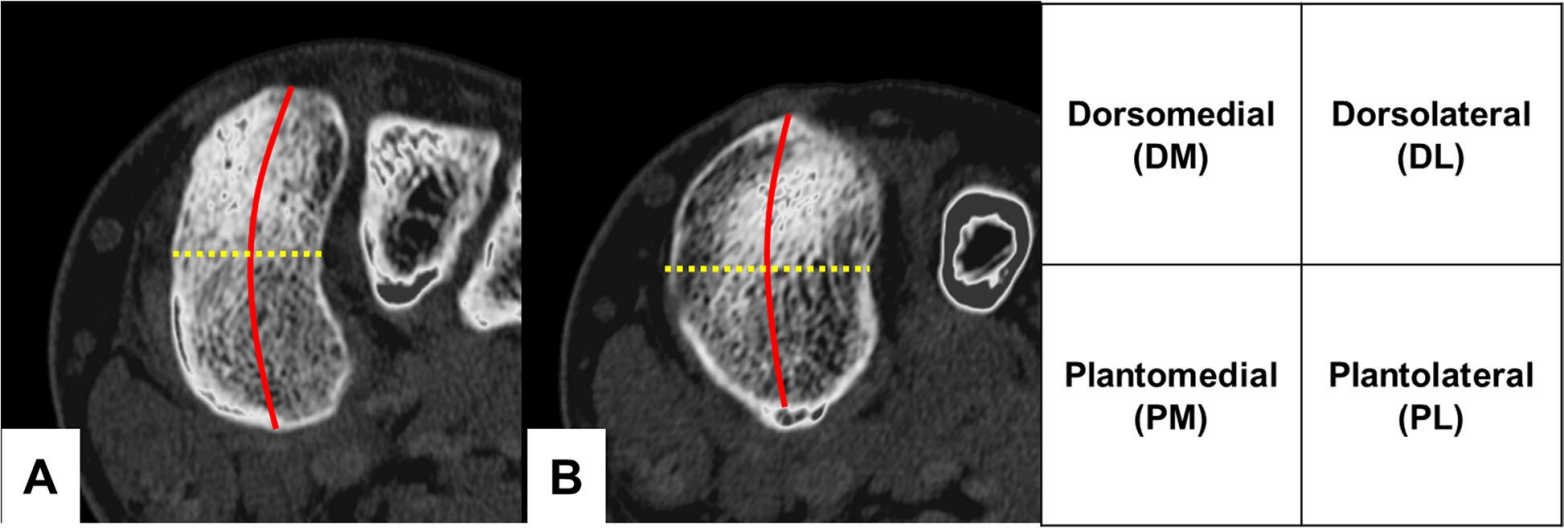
Four subdivided areas of the first tarsometatarsal joint in the medial cuneiform (A) and the first metatarsal (B) on axial CT images. Red line: line that connects the midpoint of the mediolateral width; Yellow dotted line: bisection line of the dorsoplantar height
